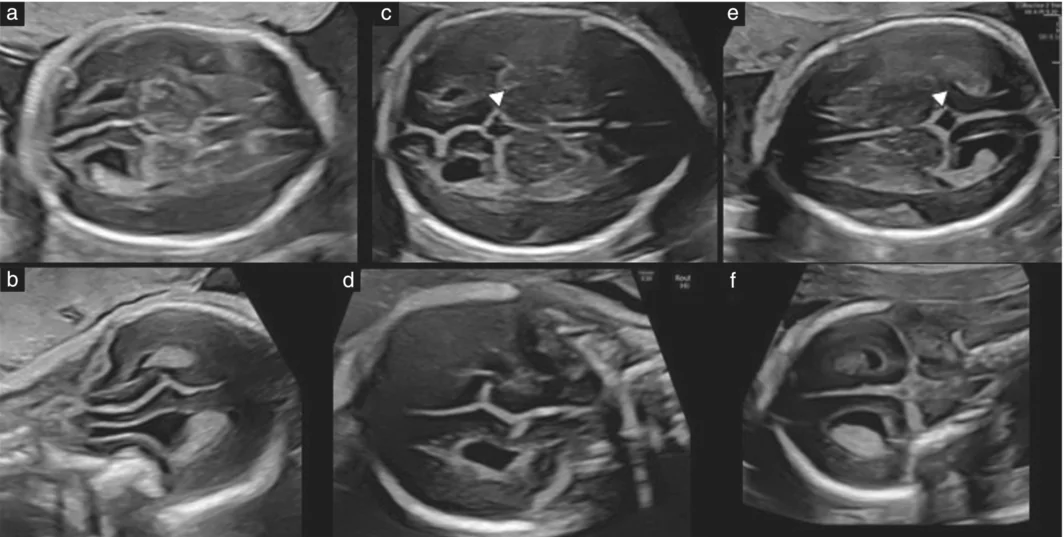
Transabdominal ultrasound images of: (a,b) fetus at 24 + 0 weeks' gestation demonstrating distortion of posterior interhemispheric fissure (IHF) in axial (a) and coronal (b) views; (c,d) fetus at 25 + 0 weeks' gestation demonstrating posterior IHF distortion in axial (c) and coronal (d) views; and (e,f) fetus at 21 + 3 weeks' gestation showing a normal posterior IHF in axial (e) and coronal (f) views. Arrowheads in (c) and (e) indicate a suprapineal pseudocyst.
